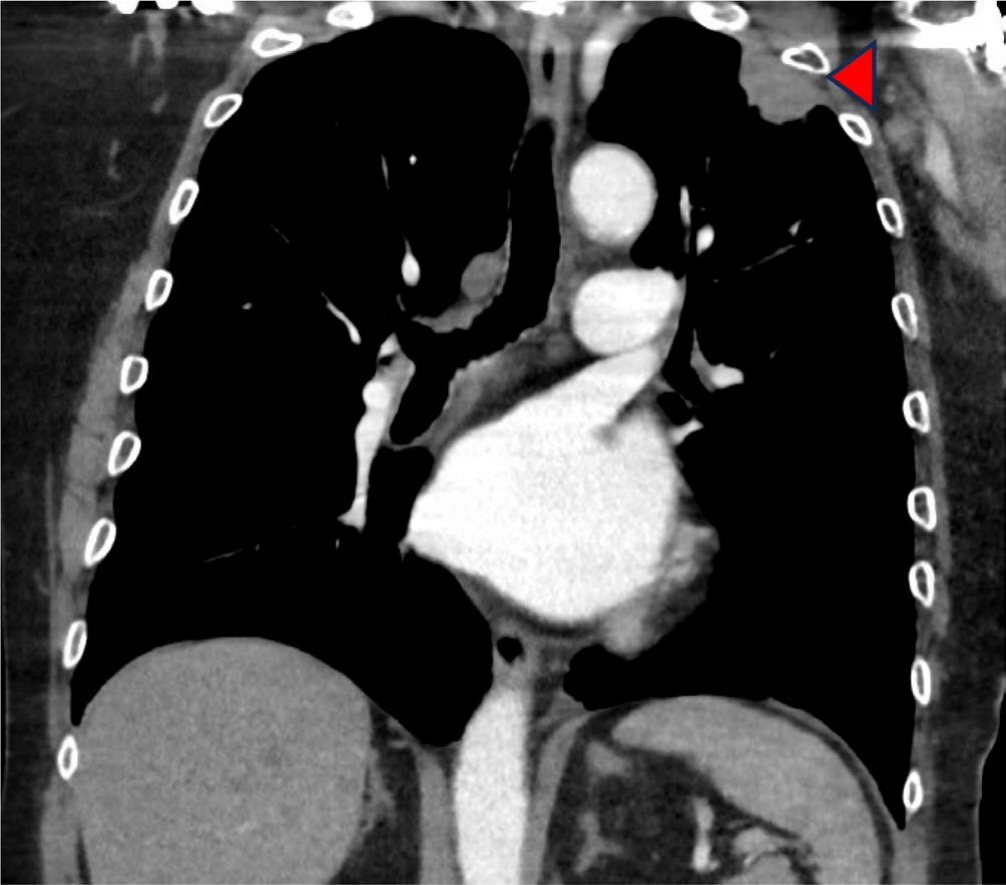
FDG PET‐CT scan showing pulmonary metastasis in the apex of the left upper lobe (see arrow) prior to treatment with pembrolizumab and pazopanib.
